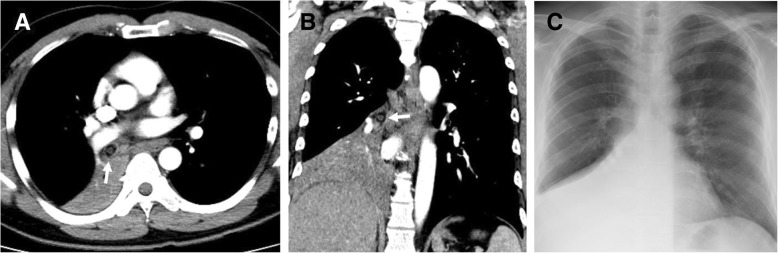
Initial radiologic findings of a previously healthy man with a solitary bronchial myelolipoma. (a and b) Initial axial and coronal chest computed tomography scan shows an endobronchial nodule of fat and a solid component in the right bronchus intermedius (arrow) combined with atelectasis of the right middle and lower lobes. (c) Initial chest radiograph shows atelectasis of the right lower lung zone
